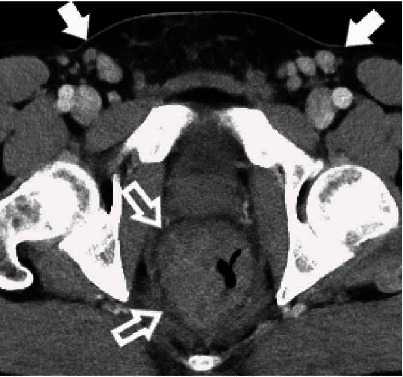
Contrast-enhanced CT in case 2 shows marked eccentric thickening of the rectal wall (open arrows) with adjacent superior rectal (not shown), external iliac (not shown), and bilateral inguinal lymphadenopathy (solid arrows). The degree of eccentric wall thickening made malignancy high on the differential diagnosis.
